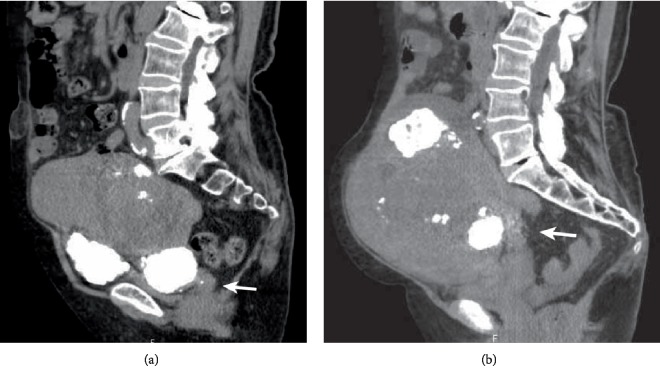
CT findings of the cervix. (a) Axial section of the lower abdomen one year before the uterine torsion. (b) Axial section of the lower abdomen at the time of uterine torsion. The cervix was elongated and moved upward compared with its position one year before (arrow).
